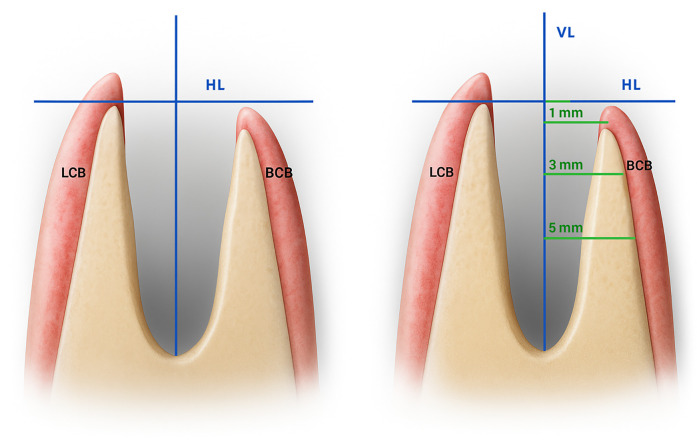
Measurement principle for socket preservation at multiple depths within the extraction site [adapted from (15)]: the distance between the lingual and buccal cortical bone is measured at 1 mm, 3 mm, and 5 mm apical depths relative to a horizontal reference line (HL) drawn on CBCT images. This line is defined by the most coronal point of the crestal bone and is oriented perpendicular to the vertical axis of the tooth. VL, vertical line (dental axis); HL, horizontal line; LCB, Lingual cortical bone; BCB, buccal cortical bone.
